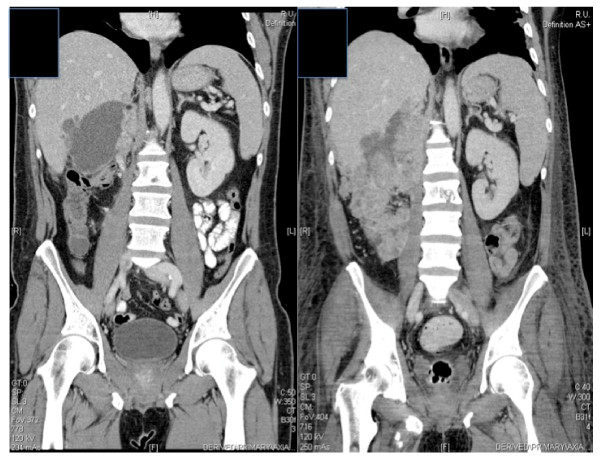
Progression of retroperitoneal tumor mass after radical nephrectomy within 4 weeks (coronal CT-images).
